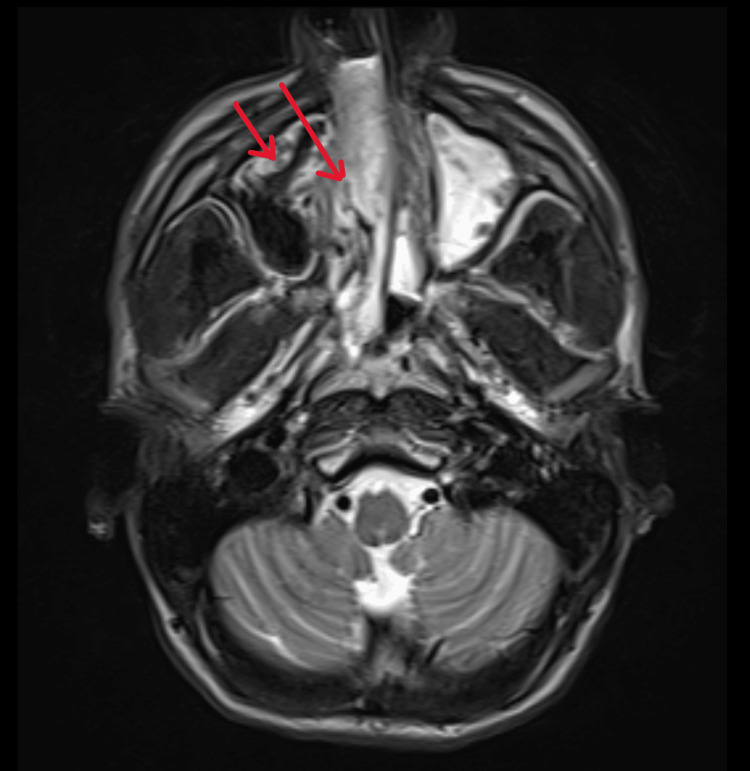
Axial T2-weighted MR image demonstrating marked T2 signal dropout (red arrows) within the sinonasal contents, consistent with dense fungal secretions. MR, magnetic resonance
